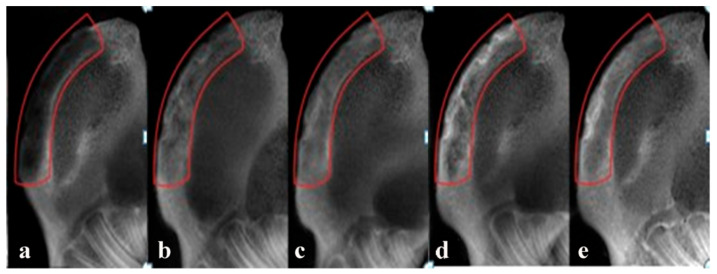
X-ray radiographs of large-sized mandibular defects repaired using different implants at week 12 post-implantation. (a) Scaffold alone; (b) BMSCs/scaffold; (c) BMSCs/bFGF/scaffold; (d) BMSCs/BMP-2/scaffold; (e) BMSCs/bFGF/BMP-2/scaffold.
